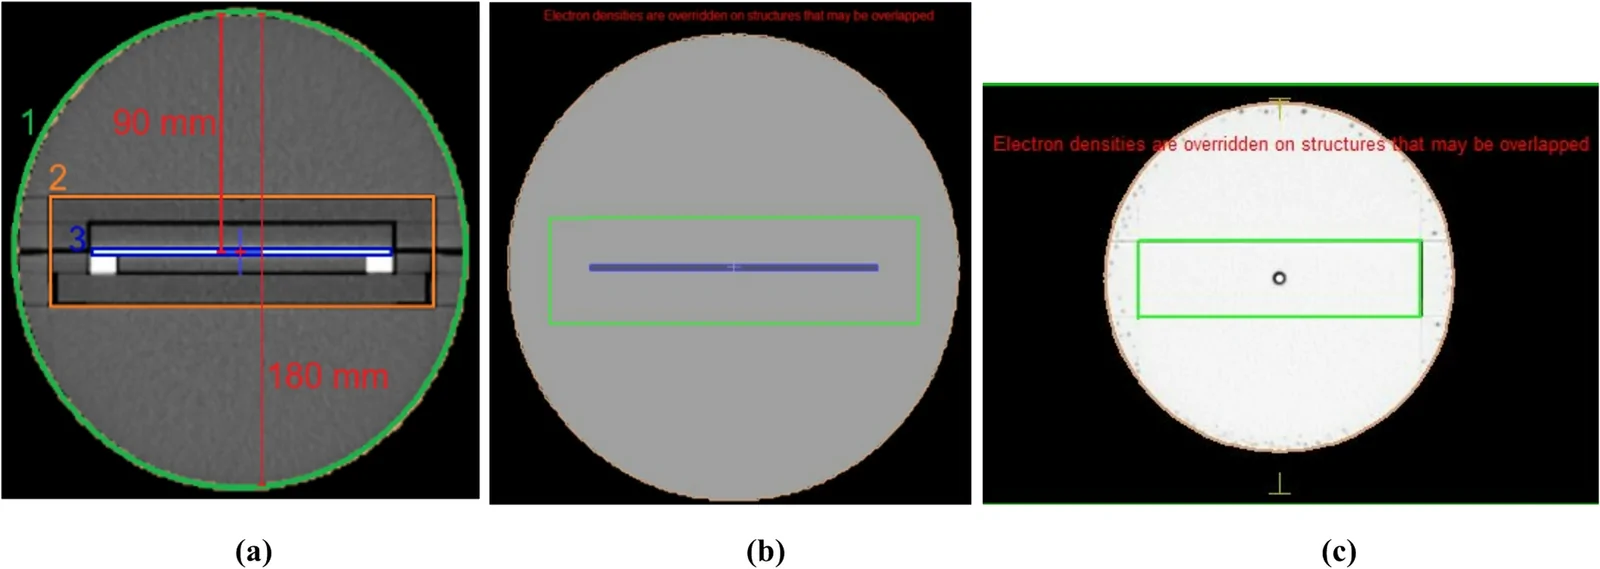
CT axial section of SRSPhan. a Dimension of delineated ABS and detector (b) The synthetic CT images of the SRSPhan with overriding Relative Electron Density (RED), (c) Ion chamber location into the inserted plug of SRSPhan
